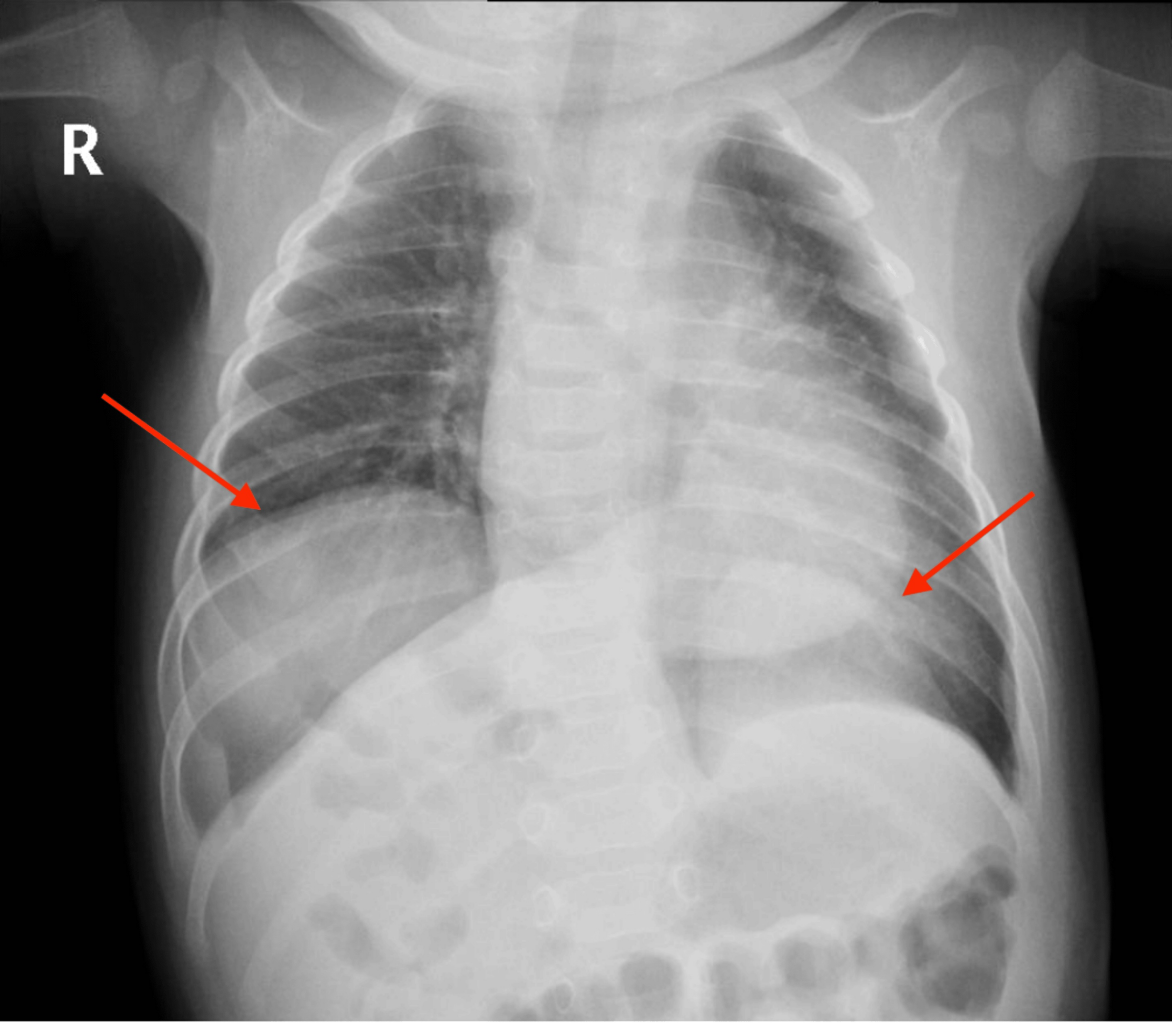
Chest radiograph showing the bilateral diaphragmatic hernia (red arrows)
